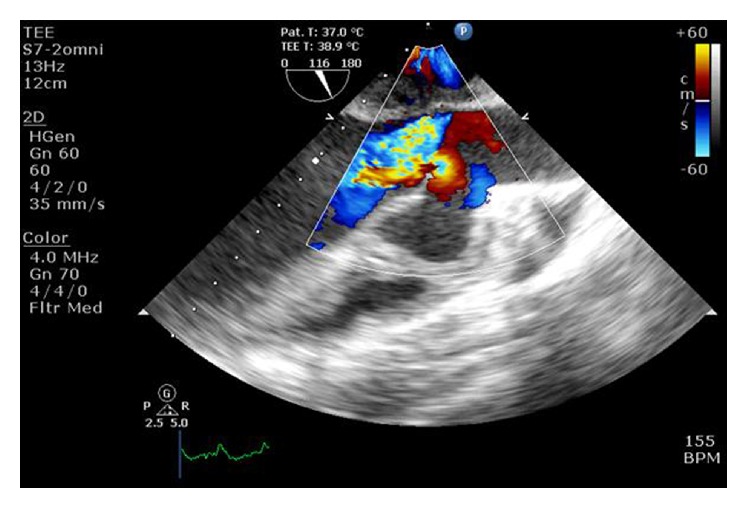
Transesophageal echocardiography^*∗*^ with color Doppler revealed mild-to-moderate aortic regurgitation due to the prolapse of the left coronary cusp into the left aneurysm of sinus of Valsalva. ^*∗*^Aortic valve long-axis view at midesophageal level.
